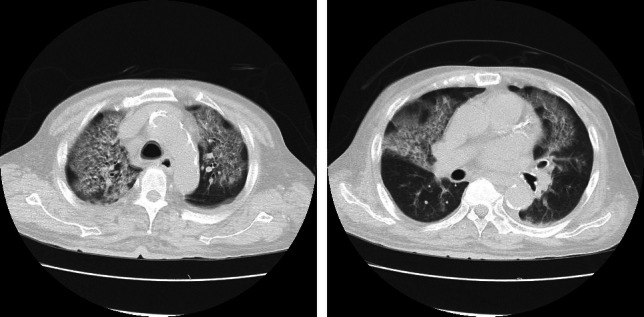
A computed tomography scan on day 3 of hospitalization shows extensive ground-glass opacities in all lung lobes
